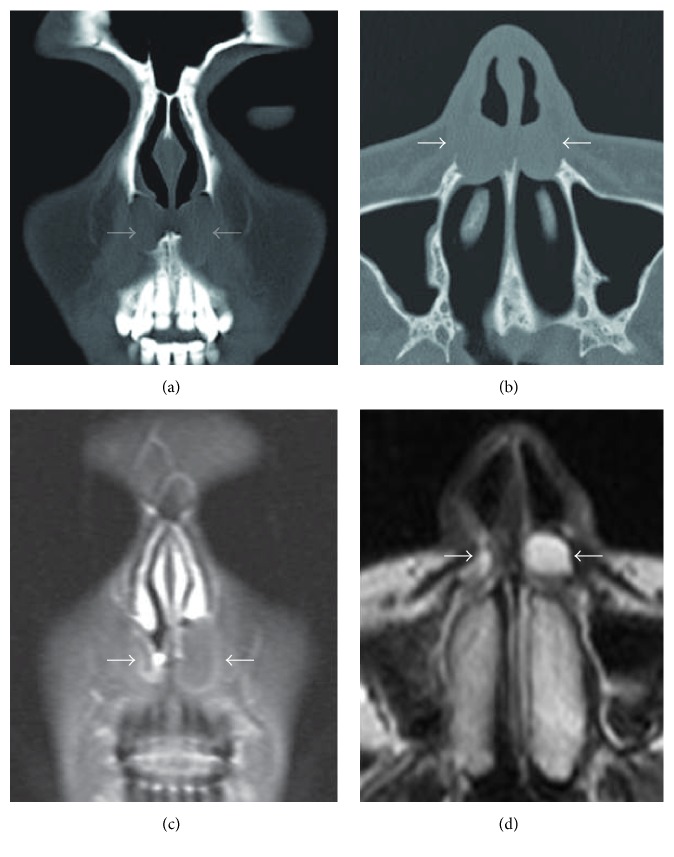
In the CT imaging of the maxillary bone, in both nasal vestibule floors, properly limited hypodense cystic lesions were observed, which were measured to be approximately 2 cm in the left and 1 cm in the right ((a) coronal view, (b) axial view). In the MR imaging performed after the antibiotherapy, at the same location, there were observed properly limited cystic lesions that did not show contrasting and looked hypointense at T1 display and hyperintense at T2 display ((c) coronal view, (d) axial view).
